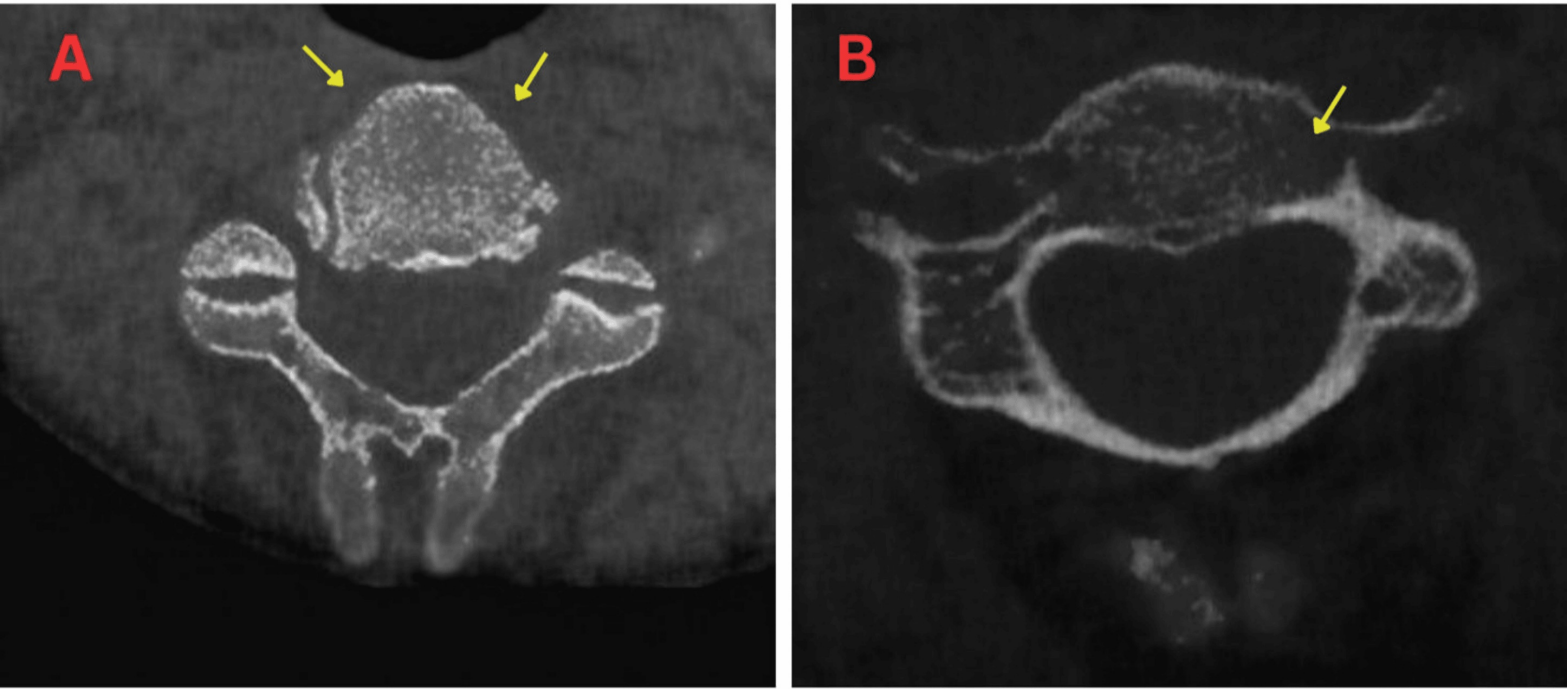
CBCT imaging showing upper cervical vertebrae grade 1. A: Mild thinning of cortical outline. B: Mild rarefaction of the trabecular pattern. CBCT: cone-beam computed tomography.
